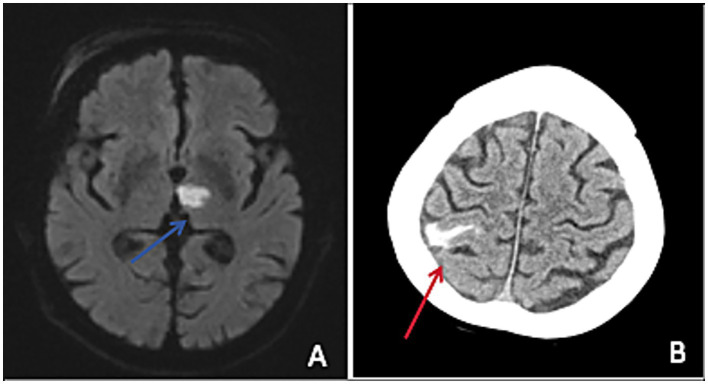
A case of HBC-3c hemorrhagic transformation following intravenous tenecteplase thrombolysis. (A) Diffusion-weighted magnetic resonance imaging (DWI) demonstrates restricted diffusion consistent with acute cerebral infarction (blue arrows). (B) Non-contrast head computed tomography (CT) shows a subarachnoid hemorrhage corresponding to hemorrhagic transformation (red arrows).
